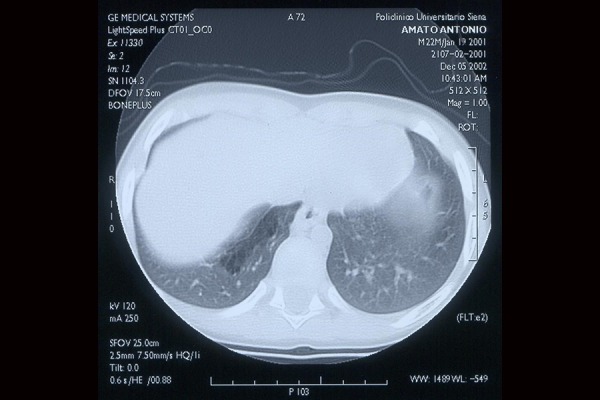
Computed Tomography: multicystic lesion of the right lower lobe.
